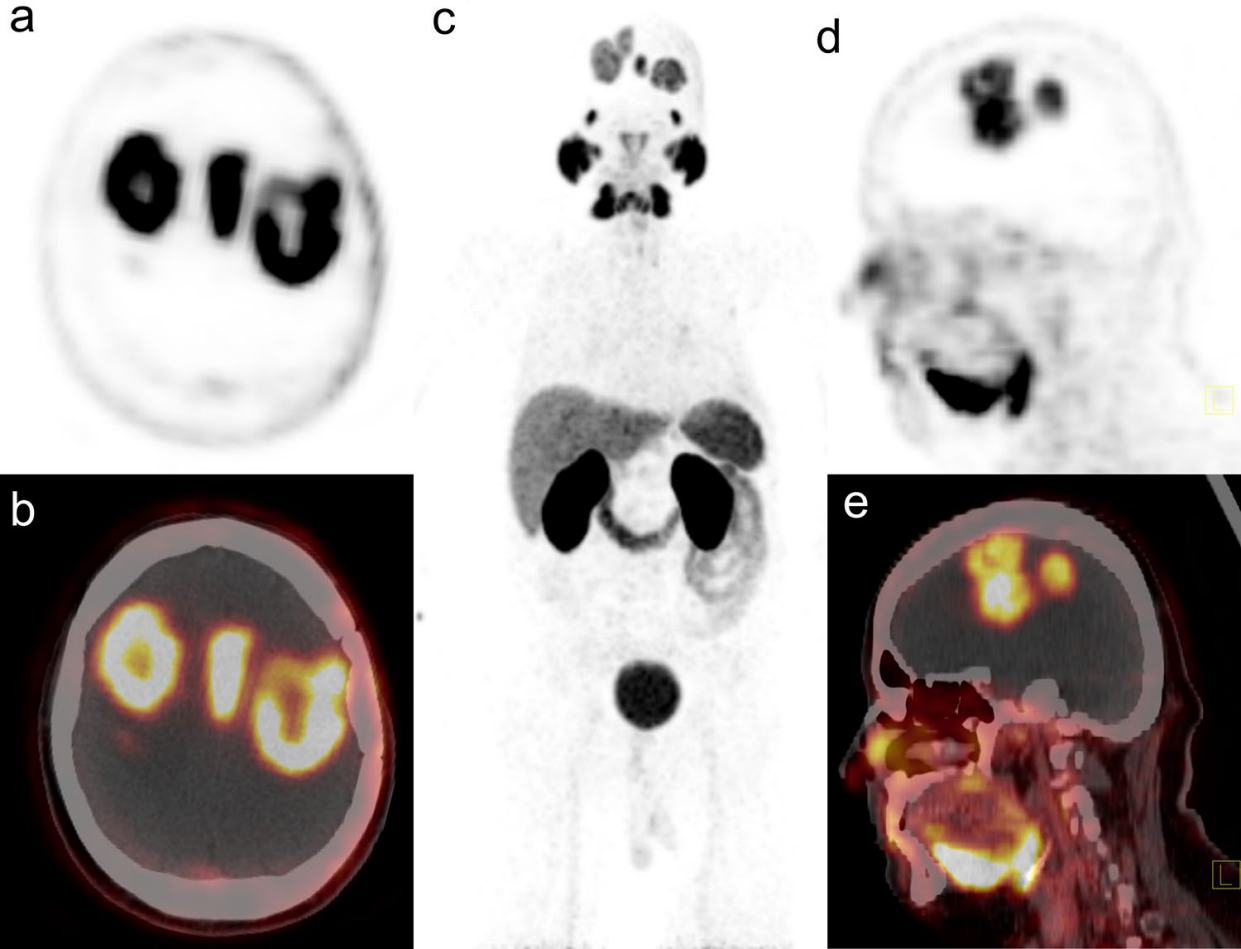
Male, 54 years, with a recurrent multiple high-grade glioma. MR performed 4 months after treatment initiation revealed a mass around the surgical cavity in the left temporo-parietal lobe and multifocal tumors in the right frontal, right parietal lobe and parasagittal area. [^68^Ga]Ga-PSMA-11 PET/CT showed high accumulation in the all tumors with the highest SUV_max_ 15.6, SUV_mean_ 7.5, TBR 156 and TLR 2.8 (a—PET transverse image, b—fused PET/CT transverse image, c—maximal intensity projection, d—PET sagittal image, e—fused PET/CT sagittal image).
